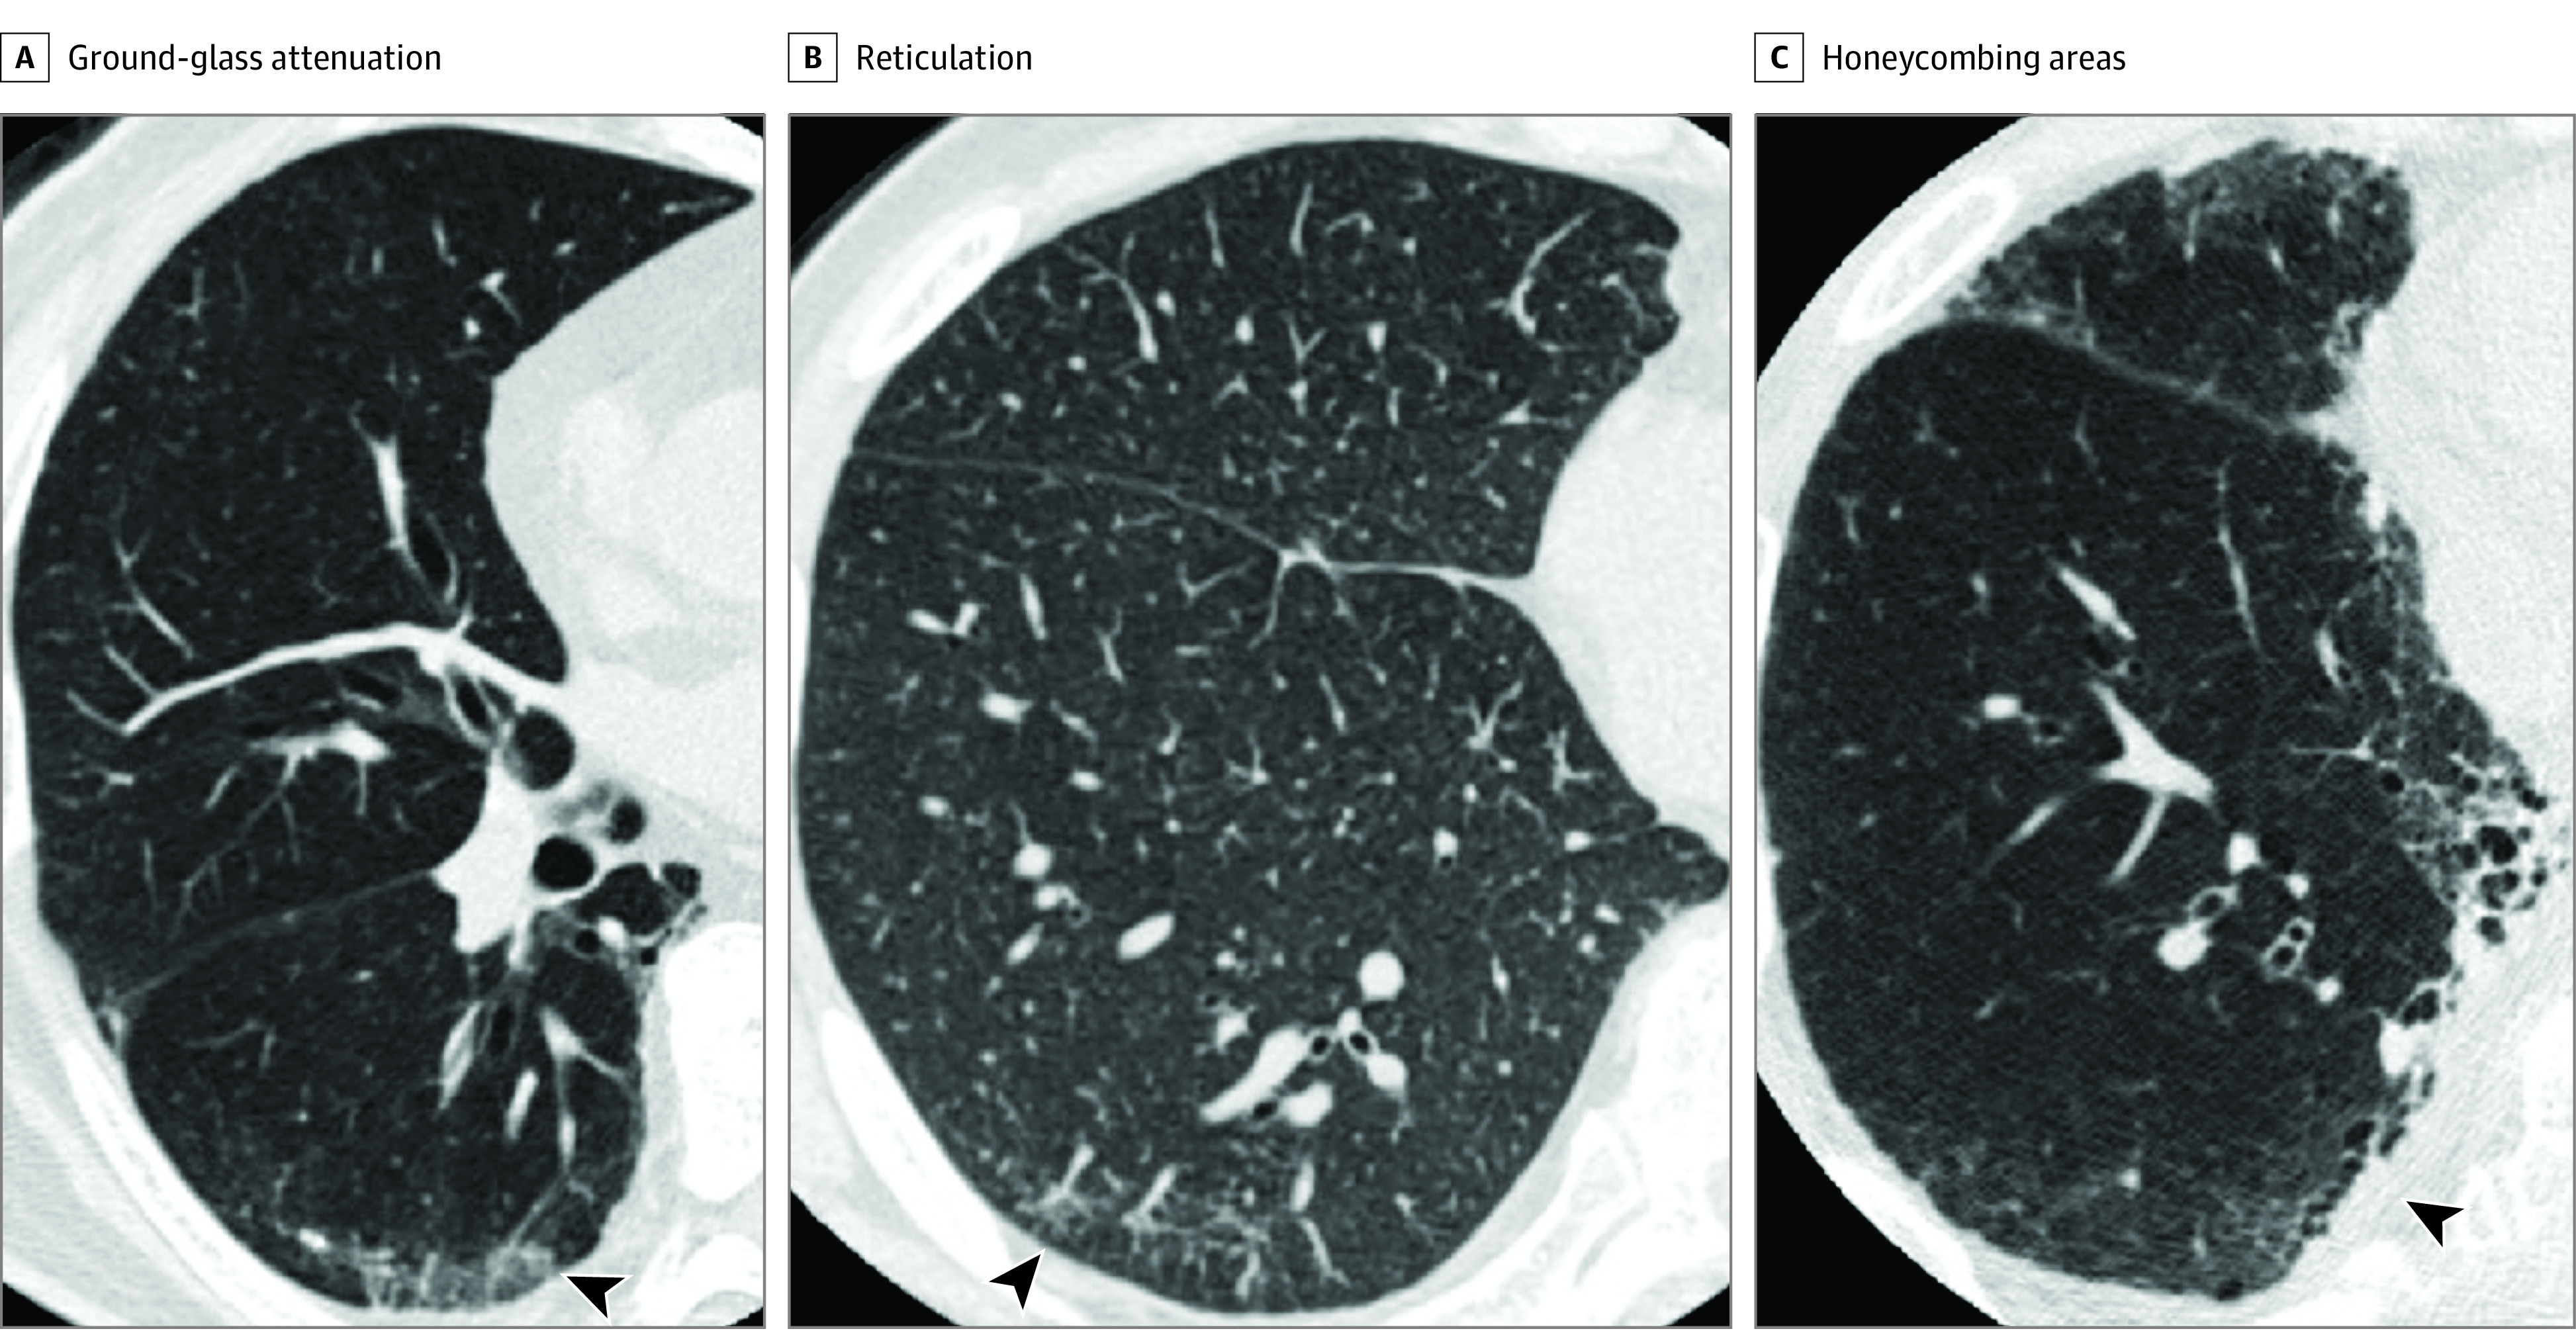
Computed Tomography Images of Interstitial Lung Abnormalities
